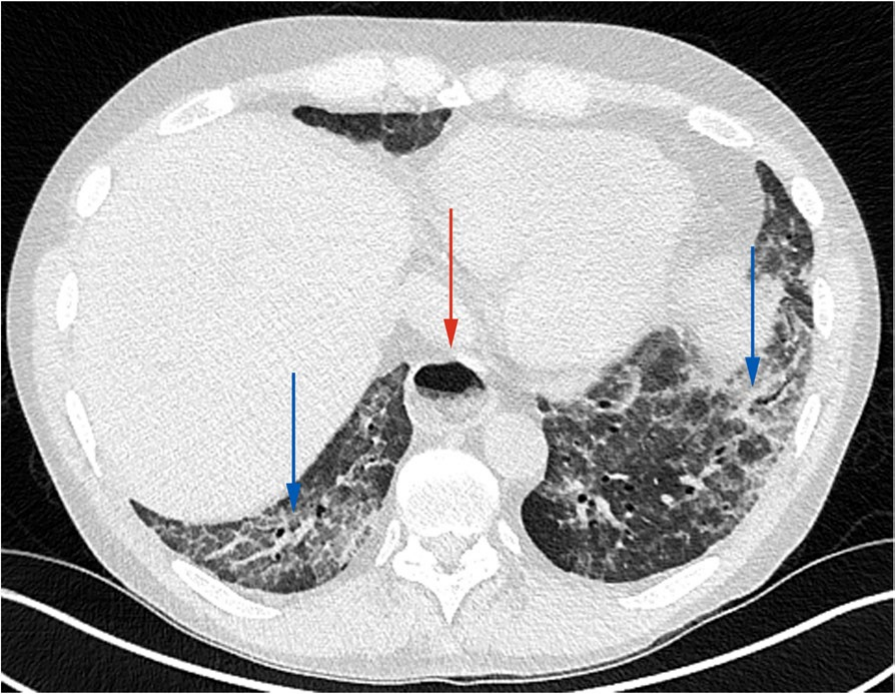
Recurrent CTD-ILD (NSIP pattern) in a 48-year-old male approximately 1 year out from lung transplant for scleroderma ILD and pulmonary hypertension. Axial CT (lung window) at the level of the lung bases shows bases shows ground glass density and traction bronchiolectasis with areas of subpleural sparing (blue arrows). A patulous debris filled esophagus is present (red arrow), consistent with esophageal dysmotility. In the setting of opacities, rising troponin and creatine kinase, and new gastroesophageal reflux, BAL with biopsy was performed which was negative for rejection and showed elevated eosinophils. The presumptive diagnosis of scleroderma-ILD recurrence was made
